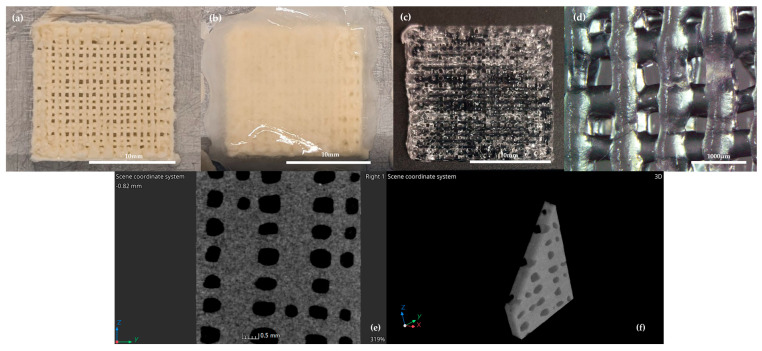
(a) Alg/Gel scaffold after the 3D–printing process onto the Peltier cell; (b) during the initial freezing and gelification process with CaCl_2_ 50mM and (c) after gelification process with CaCl_2_ 50 mM; (d) microphotograph of Alg/Gel scaffold structure at 30× magnification; CT scan images in single plane (e) and 3D reconstruction (f).
